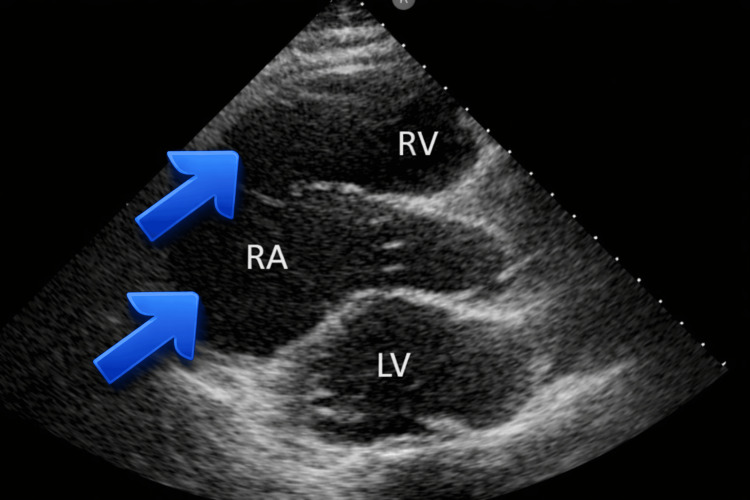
Transthoracic echocardiogram demonstrating advanced cardiomyopathy. Parasternal long-axis view shows marked right atrial (RA) and right ventricular (RV) dilation with severely reduced left ventricular (LV) systolic function, consistent with end-stage ischemic cardiomyopathy.
